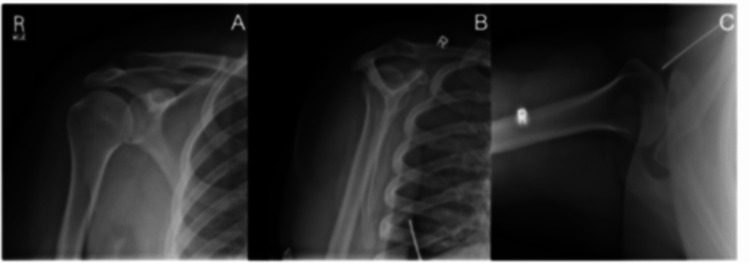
X-ray of the right shoulder showed a normal acromioclavicular joint and no acute osseous abnormalities, fractures, dislocations, or arthritic changes. (A) Right shoulder anterior-posterior view. (B) Right shoulder posterior-anterior view. (C) Right shoulder axial view.
